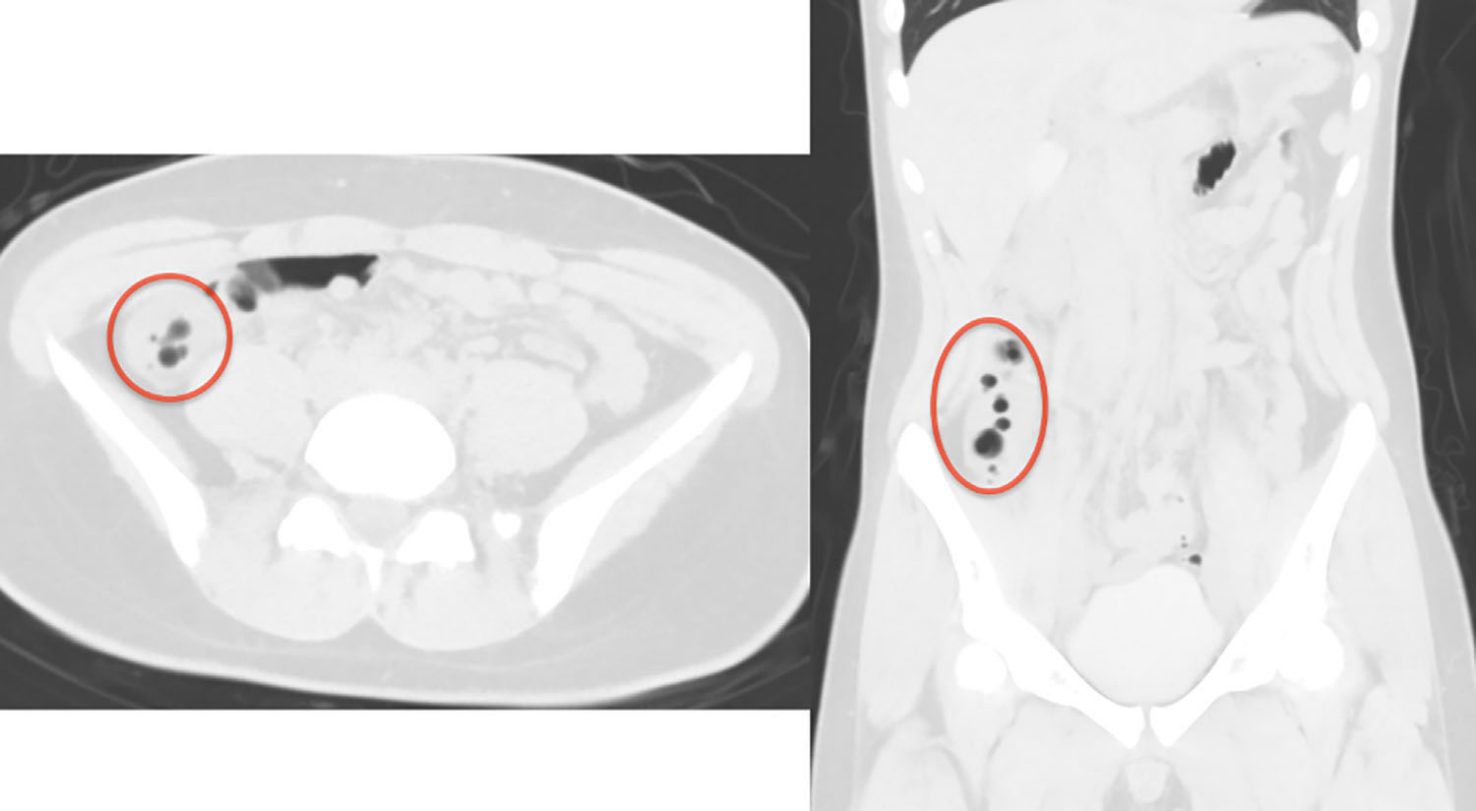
Computed tomography image (lung window) immediately after endoscopy showing findings of multiple spherical emphysematous lesions in the wall of the ascending colon (red circle).
